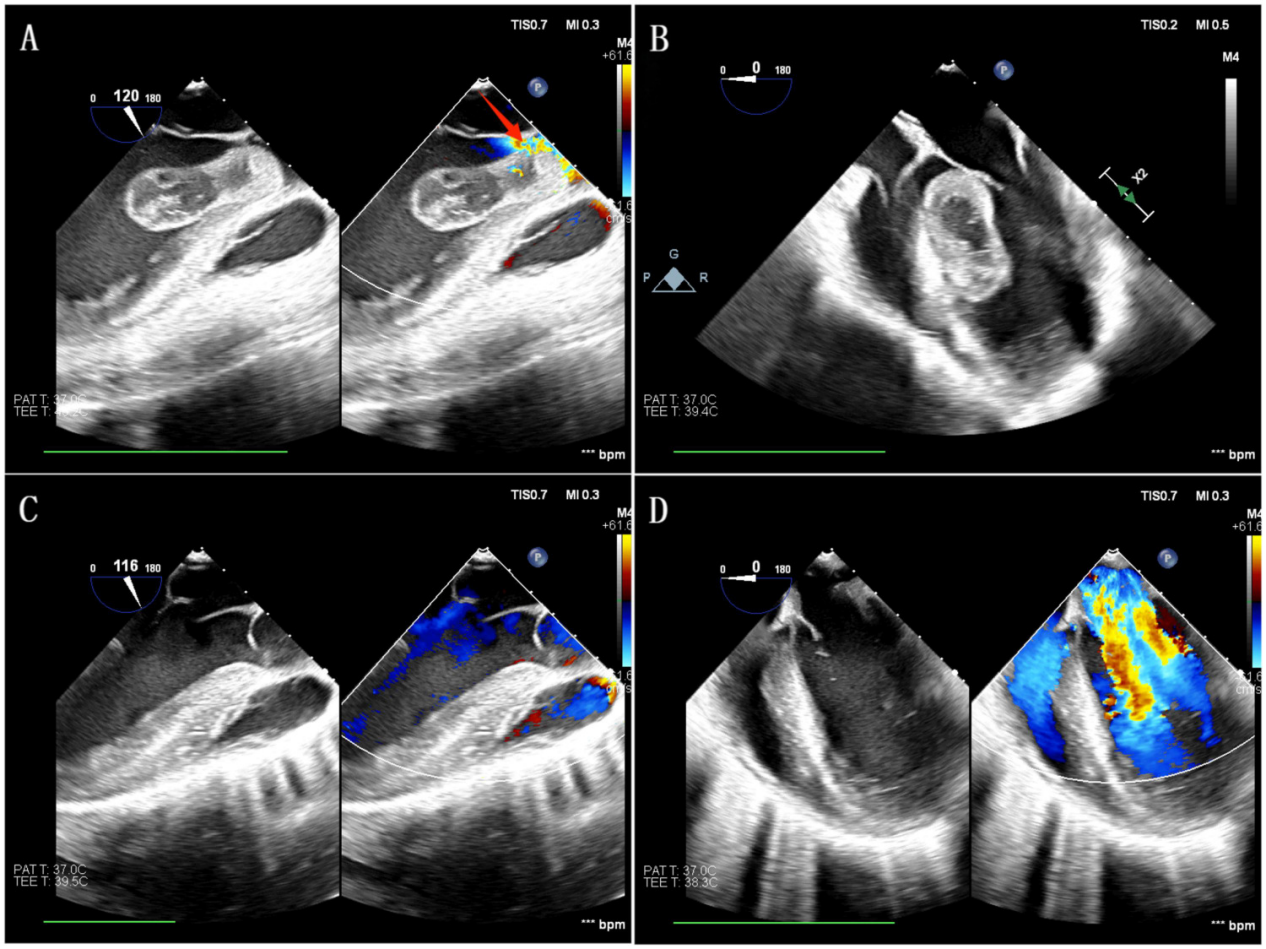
TEE findings and confirmation of complete resection. Intraoperative TEE revealed a tumor projecting prominently into the left ventricular outflow tract during systole (long-axis view), partially crossing the aortic valve and causing severe outflow tract obstruction (as indicated by the red arrow). Continuous Doppler measurements demonstrated a peak left ventricular outflow tract flow velocity of 4.94 m/s and a mean transvalvular pressure gradient of 99 mmHg, with prominent high-velocity turbulent mixed signals (A) The tumor was attached to the mid-ventricular septum via a pedicle. During diastole (four-chamber view), the mass retreated into the left ventricle and significantly obstructed the opening of the mitral valve's anterior leaflet (B) Postoperative TEE demonstrated successful resolution of left ventricular outflow tract obstruction, with complete disappearance of subaortic outflow tract color turbulence signals. The maximum flow velocity in the ventricular outflow tract decreased to 0.95 m/s, and the mean transvalvular pressure difference was 4 mmHg (C) The ventricular septum remained continuous and intact, with homogeneous surface echogenicity. The anterior leaflet of the mitral valve could be fully opened without restriction (D).
